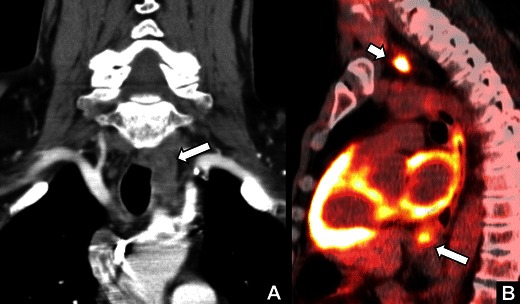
Paratracheal lymph node metastasis from oesophageal carcinoma.* A 53-year-old man with a history of heavy smoking and drinking presenting with left VCP. a Coronal contrast-enhanced CT images showed a mass in the left TE groove in the upper mediastinum in the course of the left recurrent laryngeal nerve (*arrow*). b Sagittal fused FDG-PET-CT images showed avid FDG uptake in the mass (*short arrow*) and in a thickened portion of oesophagus just below the carina (*long arrow*). The patient was diagnosed with oesophageal carcinoma with left paratracheal lymph node metastasis causing left sided VCP
